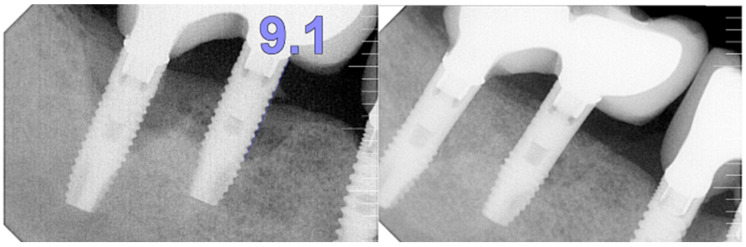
Periapical radiographies showing the re-osseointegration after using iMPACT and the Quadrant protocol. From the platform to the bone-implant contact (BIC), 9.1 mm was found.
